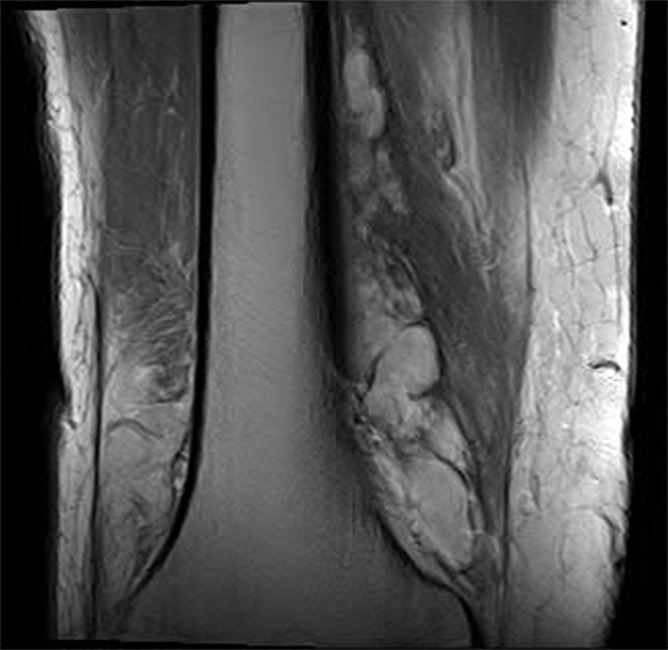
Coronal T2-weighted MRI displaying peripheral nodular enhancement along the medial aspect of the distal femur likely reflecting hematoma measuring 13.4 × 4.6 × 3.0 cm. MRI, magnetic resonance imaging.
